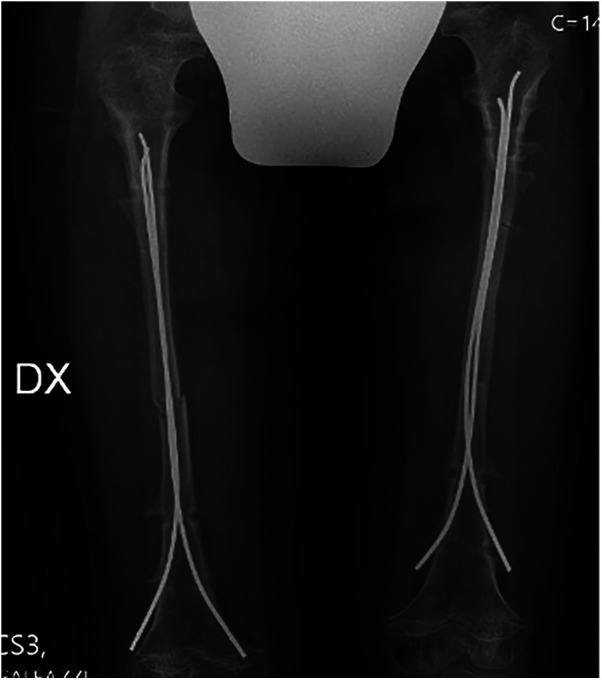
Postoperative radiograph after TENs placement following bilateral femur fracture.
